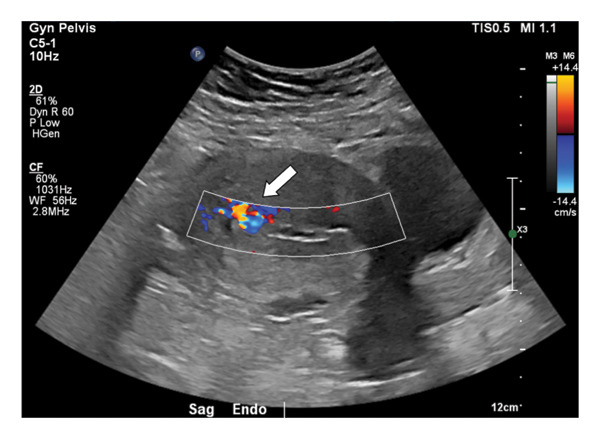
Transvaginal ultrasound demonstrating intrauterine color Doppler flow concerning for hypervascular RPOC or AVM.
